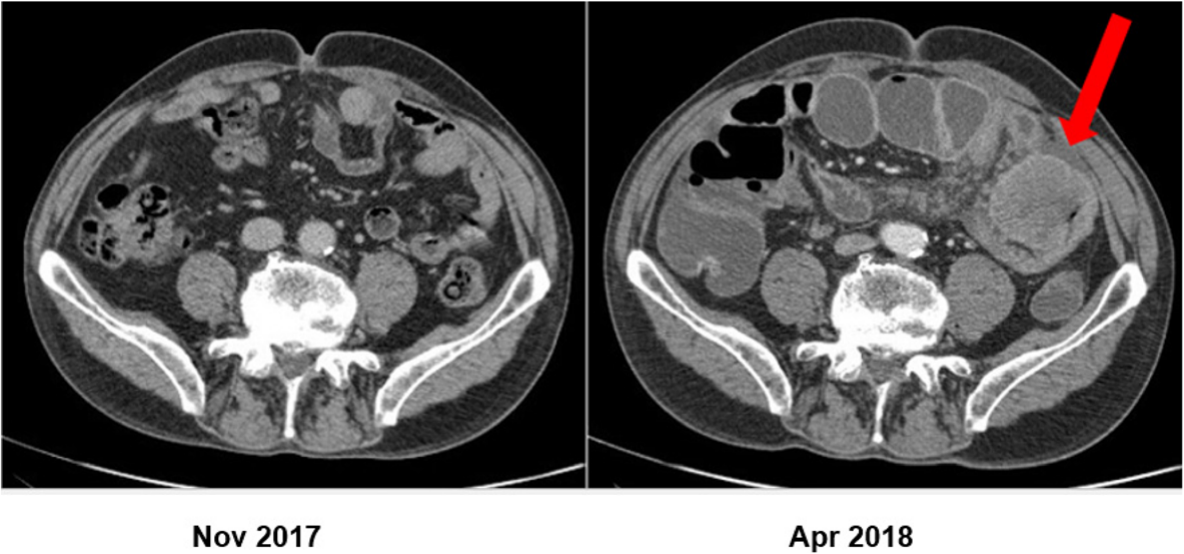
Comparative CT scan of the abdomen in November 2017 and April 2018 with new developed intraabdominal metastasis and small bowel dilatation
